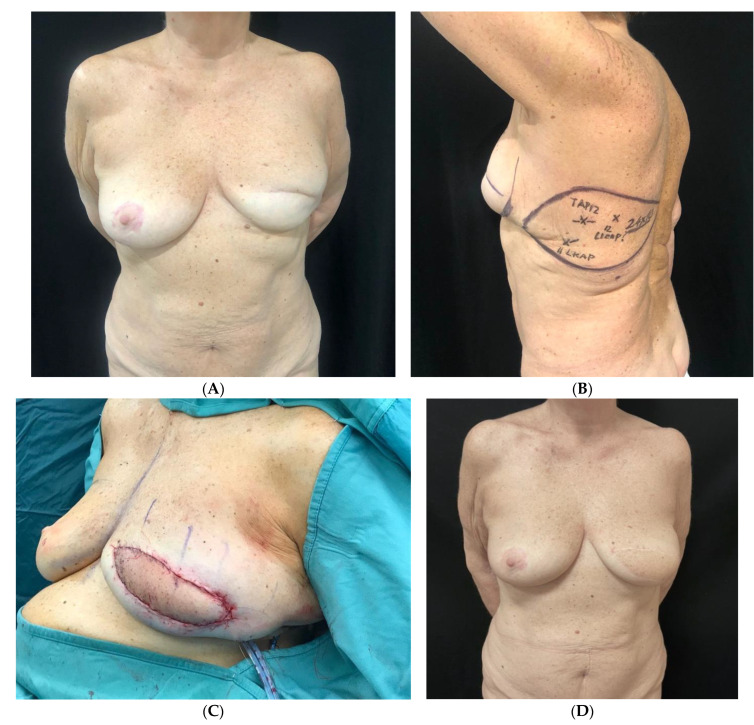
Patient planned for delayed left breast reconstruction with TDAP flap following previous skin sparing mastectomy (SSM) and submuscular tissue expander placement. (A) Preoperative view. (B) Preoperative markings of the skin paddle and TDAP perforators identified at ultrasound. (C) Intraoperative view following flap inset. (D) Postoperative view after 3-year follow-up.
